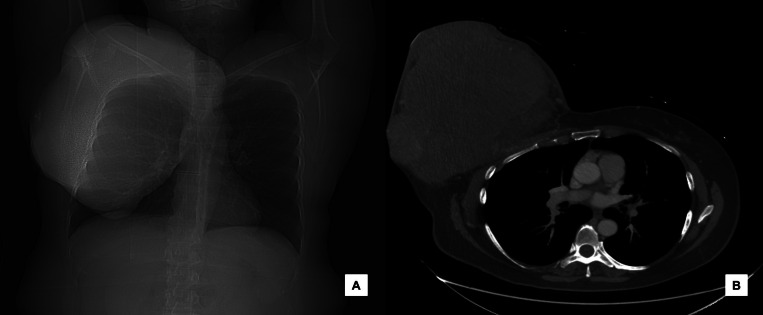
(A) Scout image of the thorax demonstrating a large mass occupying the entire right breast and (B) Axial contrast-enhanced CT image of the chest demonstrating a lobulated mass with heterogenous enhancement.
